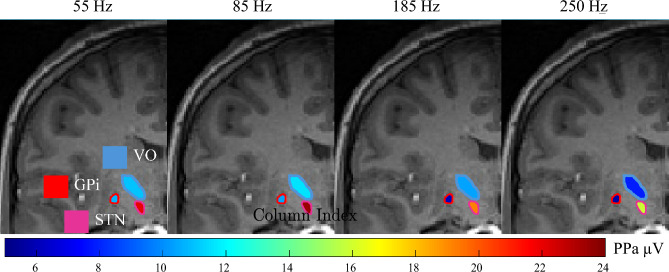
The propagation of GPi stimulation in the GPi (red outline), VO (blue outline), and STN (pink outline) regions across different stimulation frequencies. The fill colors represent the PPa of EPs detected in these regions. VO and GPi PPa start at approximately 10 $$\mu V$$ at 55 Hz and gradually decrease to around 6 $$\mu V$$ as the stimulation frequency increases, becoming darker in color. In STN, the initial PPa is red, gets darker at 85 Hz, and then shifts back to orange and yellow with further increase in stimulation frequency. The MRI image and region localization is estimated based on neuroimaging data from subject S13.
